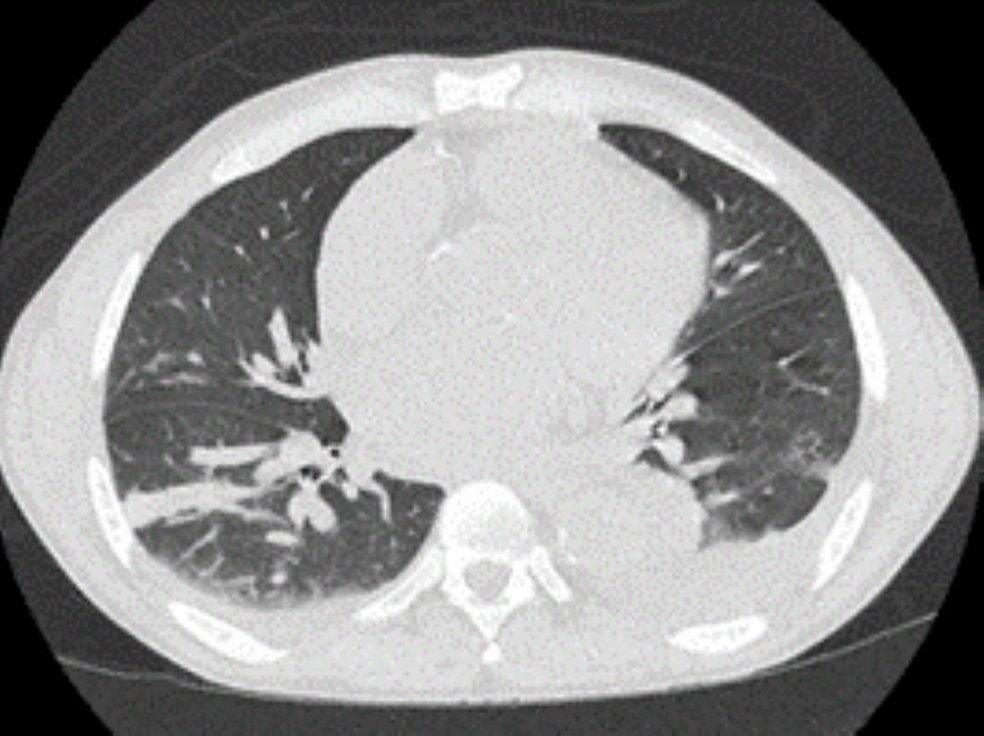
A CT scan of the chest without contrast performed after the pericardiocentesis shows small bilateral pleural effusions, left larger than right.
